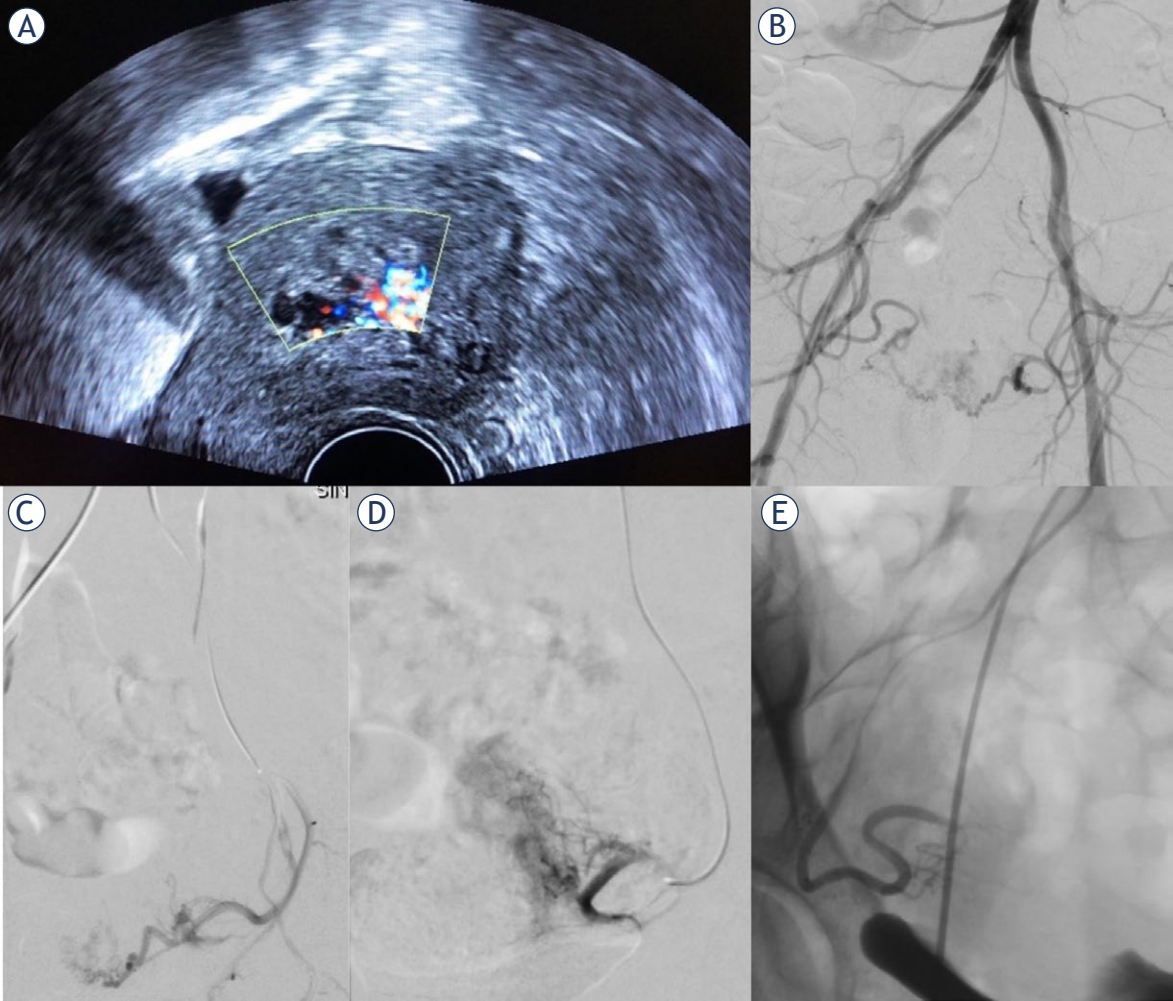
(A) Transvaginal ultrasonography in a 34-year-old female with vaginal bleeding after spontaneous termination of pregnancy at 12 weeks of gestation. Color Doppler ultrasound showed a 45 x 30 mm mass in the uterus with increased vascularity suggestive of retained products of conception. (B) Pelvic arteriogram demonstrating numerous spiral arteries in the uterus fed by both right and left uterine arteries, confirming the diagnosis of RPOC. (C) Left uterine arteriogram in the same patient before prophylactic embolization with absorbable gelatin sponge particles showing numerous spiral arteries. Postembolization left (D) and right (E) uterine arteriogram demonstrating successful embolization. Subsequently, surgical resection of retained products of conception was successfully performed.
